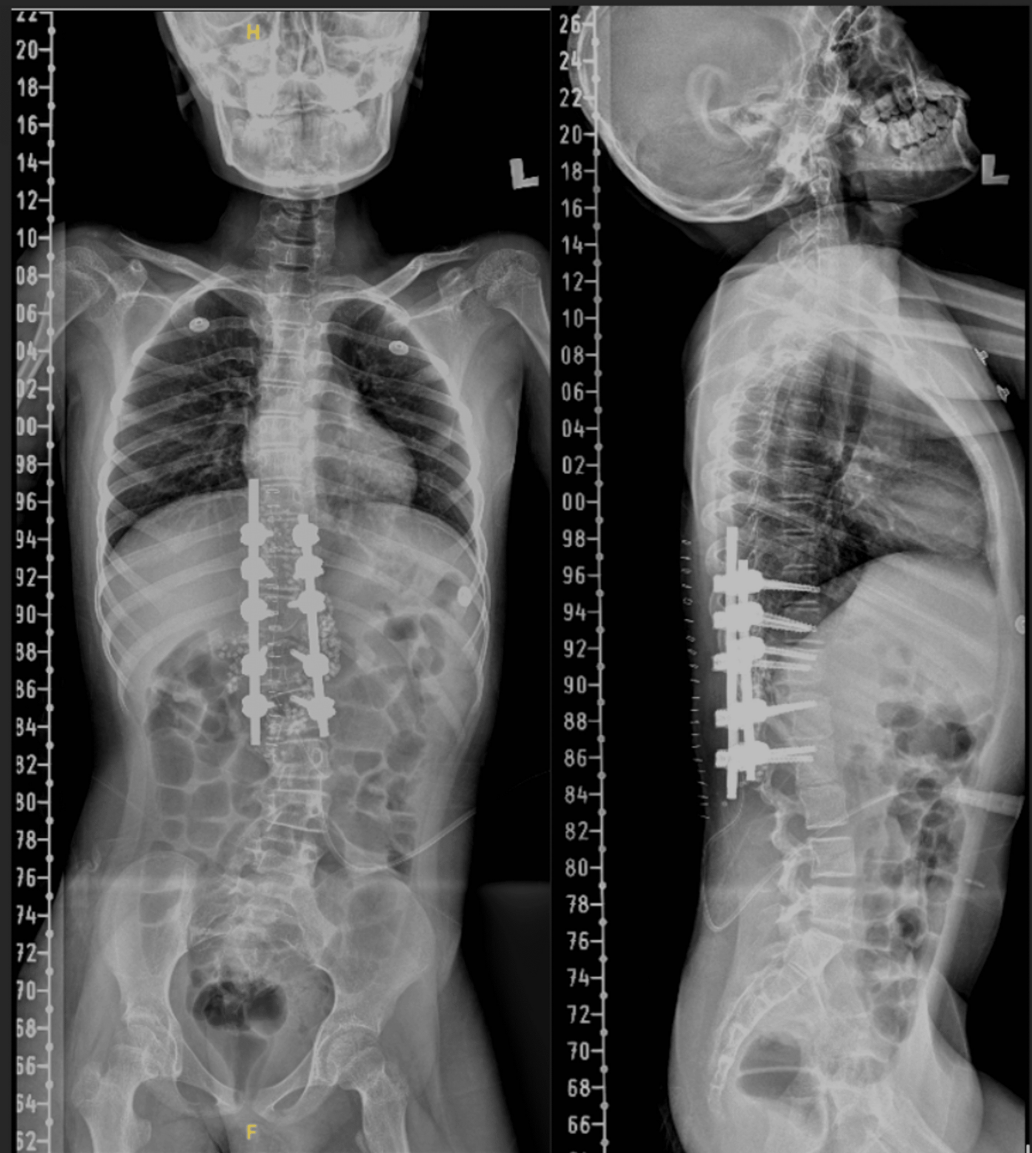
Postoperative X-rays of the whole spine depicting that the spinal deformity has been well corrected with the absence of the T12 vertebra.
